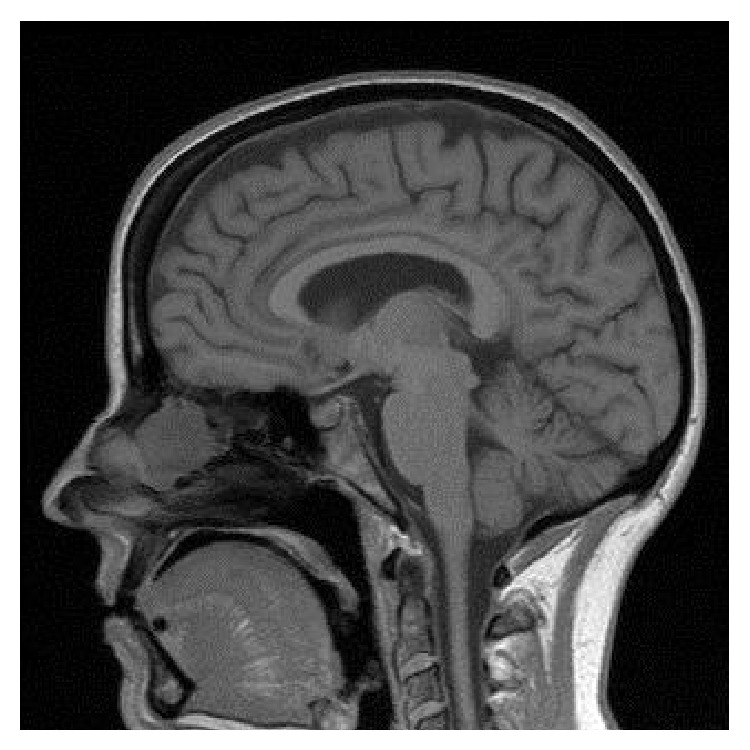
T1-weighted sagittal MRI of the nasal cavity mass.
